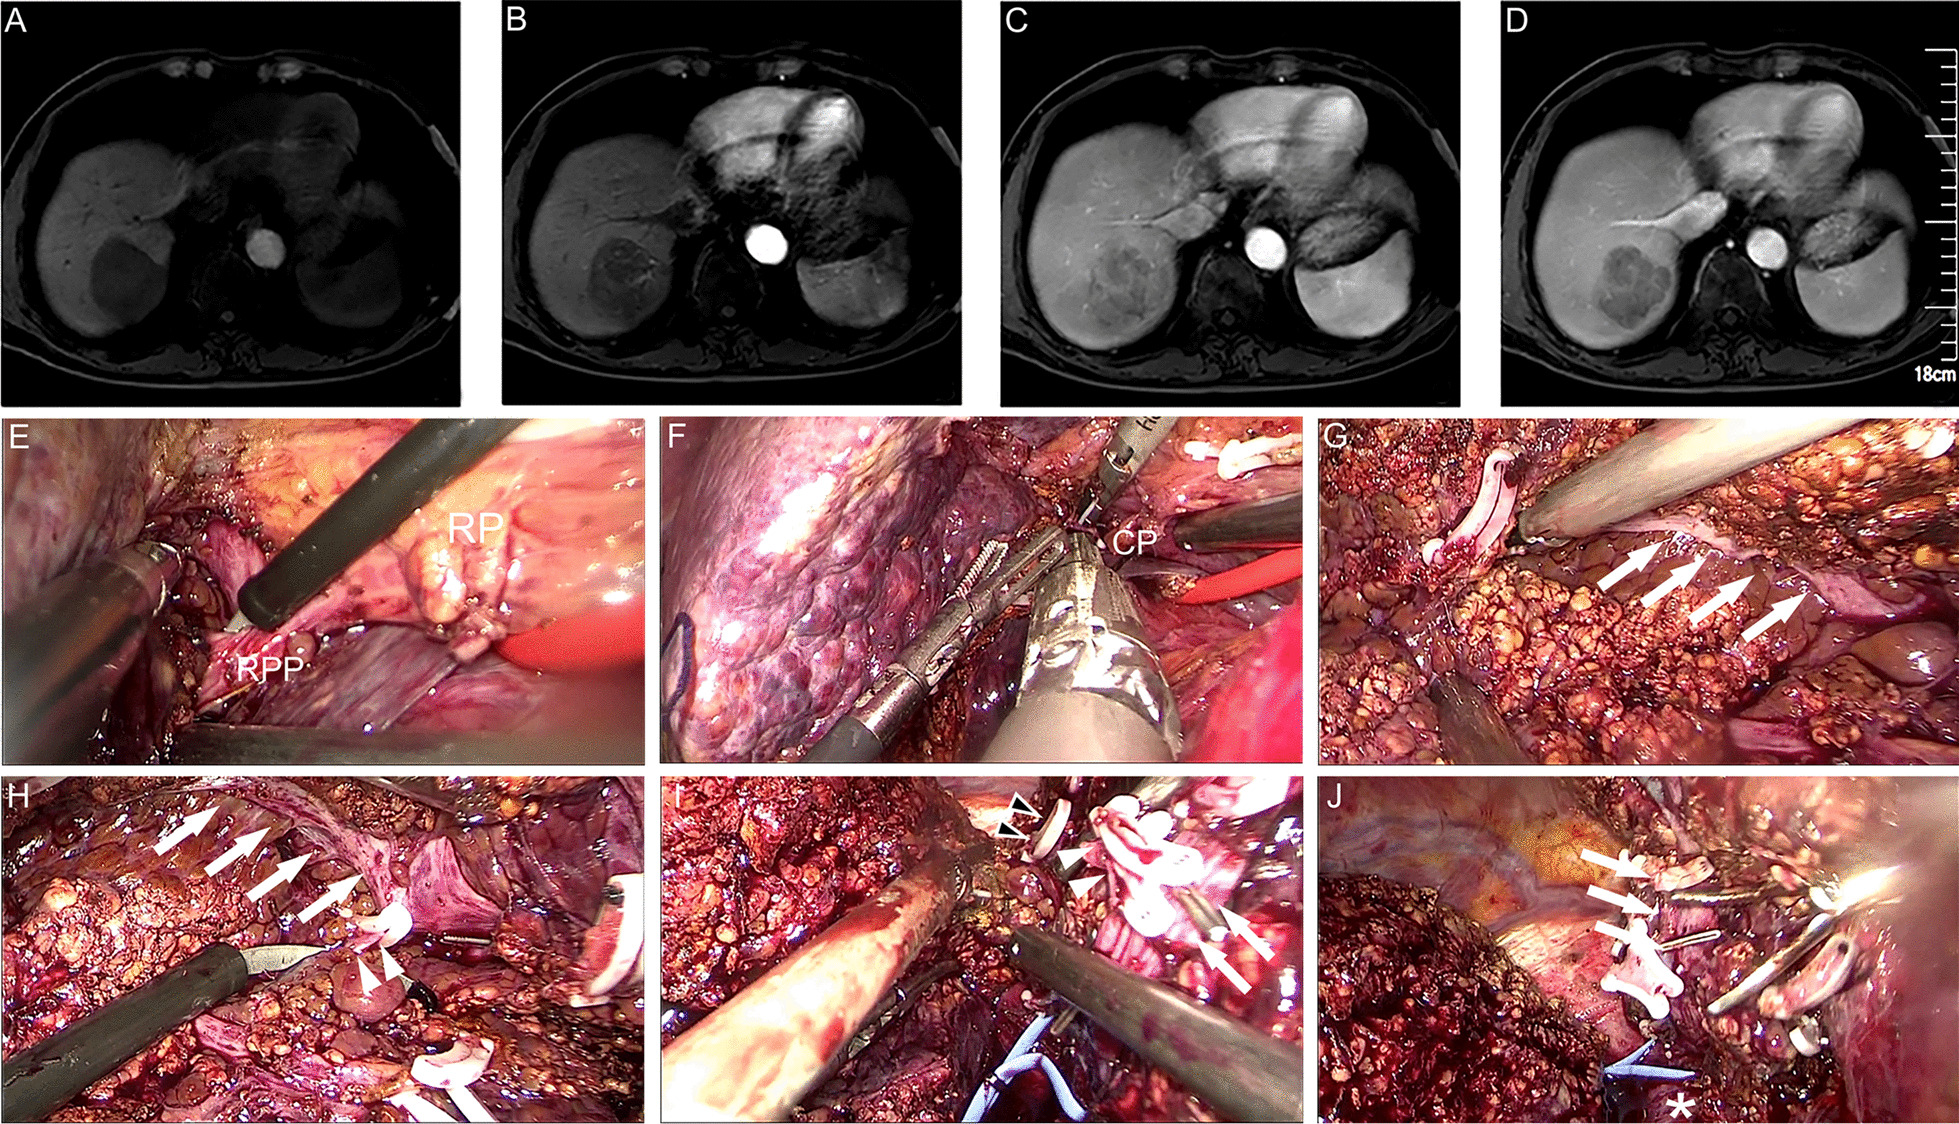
Dorsal approach with Glissonian approach in laparoscopic right posterior hepatectomy (LRPH). Preoperative MRI showed HCC (a–d) and intraoperative key view during procedure (e–j): e to isolate RPP; f to transect liver parenchymal of CP through dorsal approach; G, to transect liver parenchymal between RHV and IVC; h to isolate S6HV; i to isolate S7HV; j the right posterior of liver was transected, and RHV was clearly shown. White arrows, RHV; White arrowheads, S6HV; black arrowheads with white edge, S7HV; white asterisk, IVC
